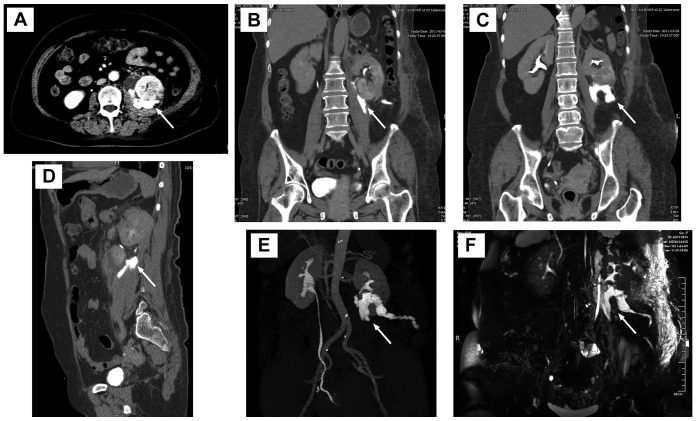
Postoperative imaging showing urinary fistula. (A–E) Urinary fistula (arrow) was detected by CT urography. (F) Urinary fistula (arrow) was detected by MR urography.
